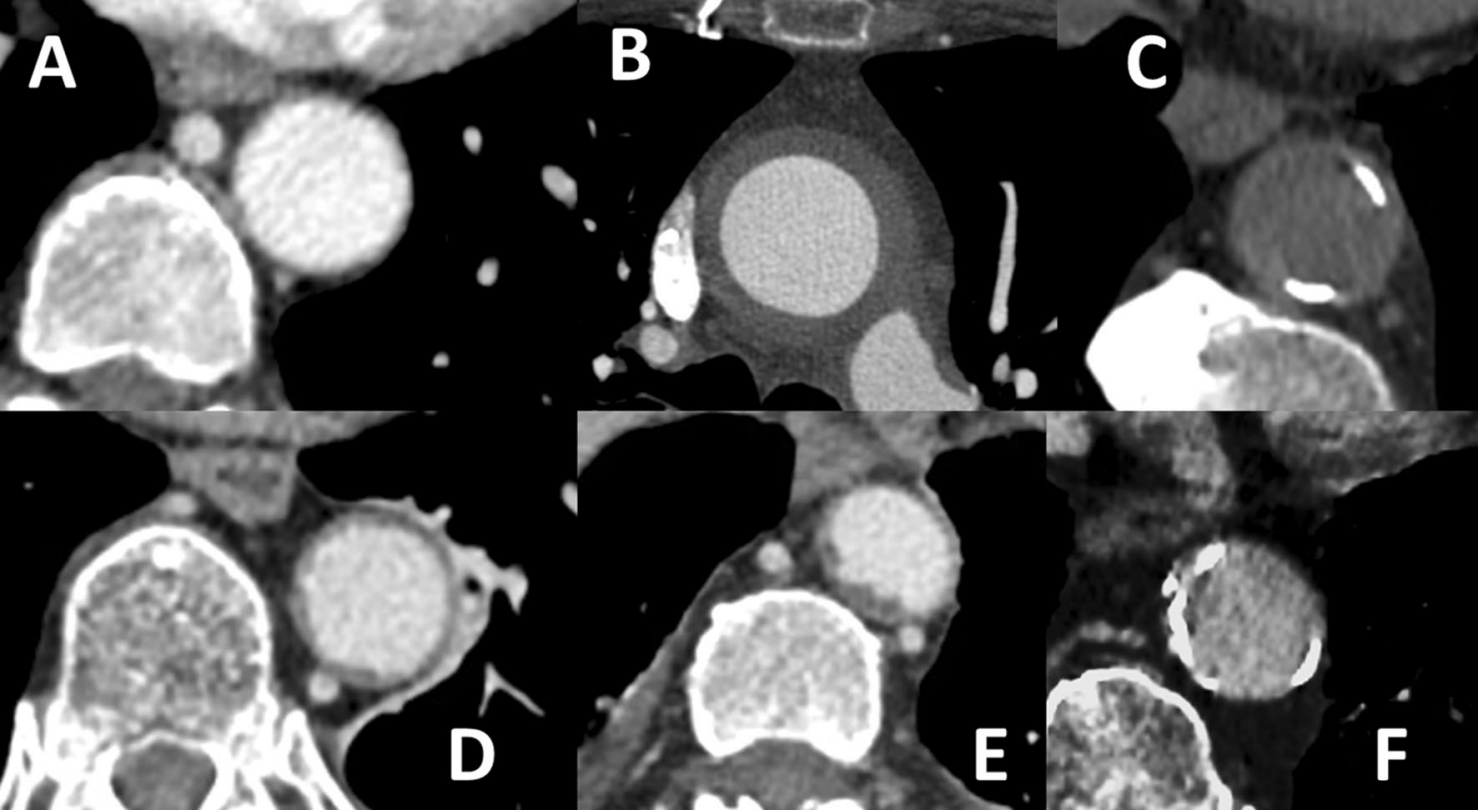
Aortic evaluation with and without contrast enhanced computed tomography (CT); (A) Normal aorta, (B) Aortitis, (C) Aortic calcification in non-enhanced CT, (D) Atheroma grade 1, (E) Atheroma grade 2, F: grade 2 mixed atheroma with calcification.
